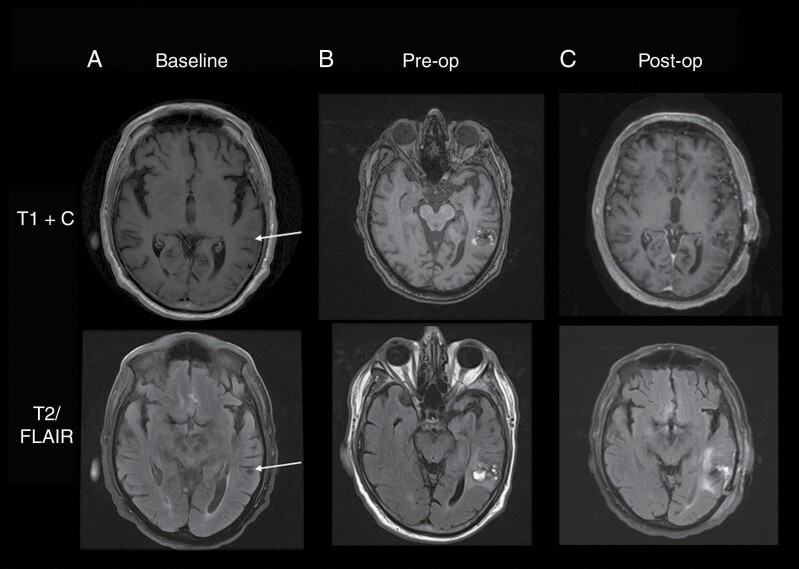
Axial T1-weighted contrast enhanced (T1 + C) and T2/FLAIR images for the patient described in the case example. (A) Images at the time of initial brain metastasis development (visible as only a very faint dot of increased signal on the T1 + C image, (B) at the time of progression approximately 1 year post-SRS treatment, and (C) postoperative imaging.
